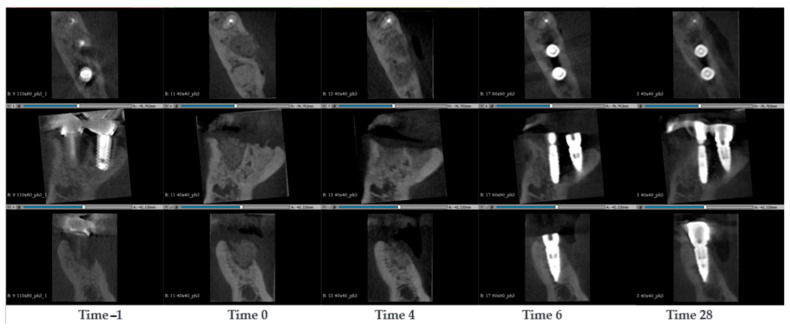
CBCT images of a 59-year-old woman with bone resorption between two dental implants. The implants were removed, and the alveolar bone void was filled with SmartBone^®^ microchips. The images show the progression of bone healing over time, from pre-surgery (Time −1) to 28 months post-surgery (Time 28).
